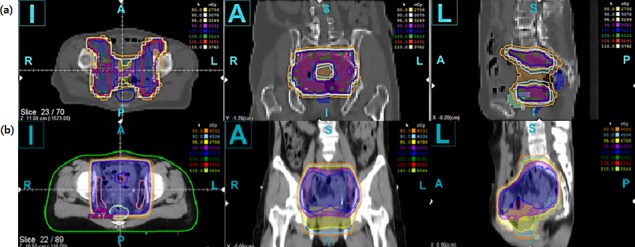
CT slice of IMRT (a) and cRT (b) radiotherapy plans with isodose lines (80%, orange; 95%, yellow; 100%, blue; 103%, green). Note that small bowel and rectum in IMRT plan are spared. (a) IMRT, Intensity-modulated Radiotherapy. (b) cRT, Conventional Radiotherapy.
